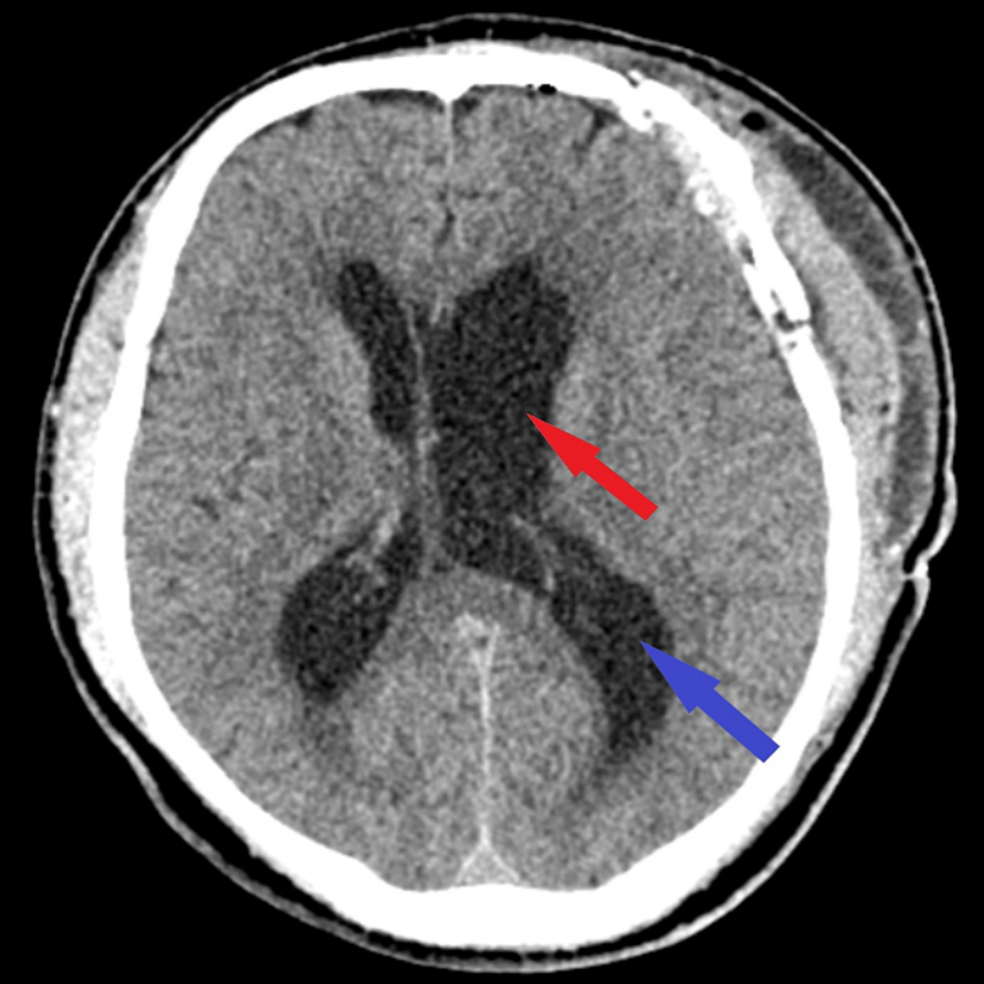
Postoperative CT scan The axial section of the CT scan performed postoperatively highlights total tumor resection (red arrow) as well as an enlarged left lateral ventricle (blue arrow)
